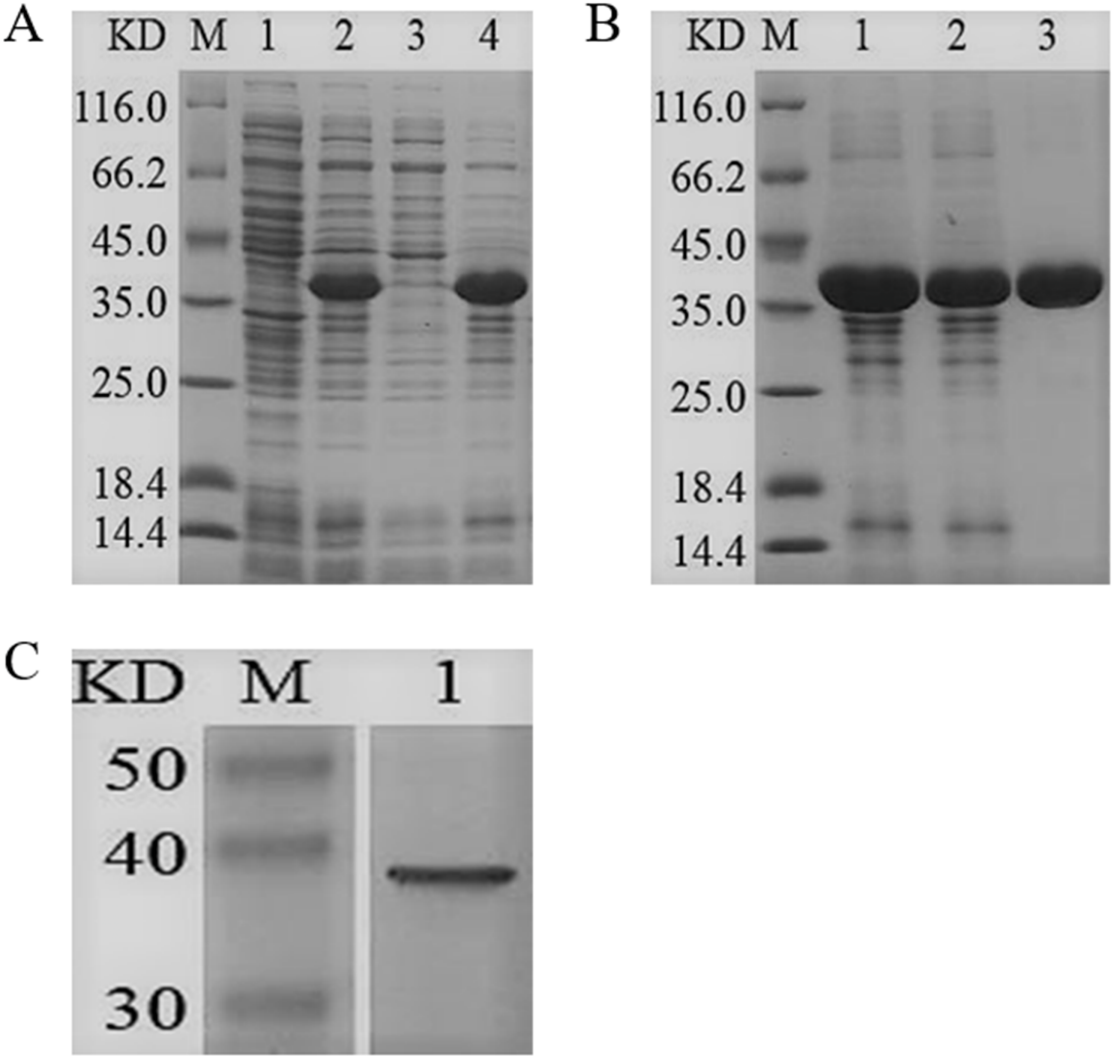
SDS-PAGE and Western blotting analysis of the purified recombinant YgjD. M denotes the middle-ranged protein marker. (A) When the recombination strain was induced for 4 h by IPTG at 37 °C, 20 µL of the culture supernatant was collected. The culture supernatant mixed with the 6× loading buffer were boiled and then determined by SDS-PAGE as shown in band 2 with the un-induced culture supernatant as control (band 1); When the induced cells were cracked by ultrasonic, the supernatant and the precipitation were analyzed by SDS-PAGE (band 3 and 4). (B) The solubilized inclusion body and dialysis solution were measured by SDS-PAGE (band 1 and 2); The purified YgjD by Ni-ID Sepharose CL-6B column was determined shown in band 3. (C) Western blot analysis of the purified YgjD is shown in band 1.
